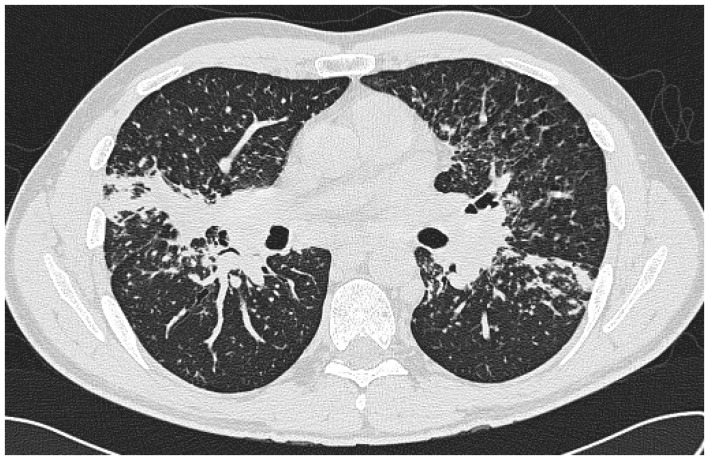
CT scan of SAIA with widespread consolidation.
